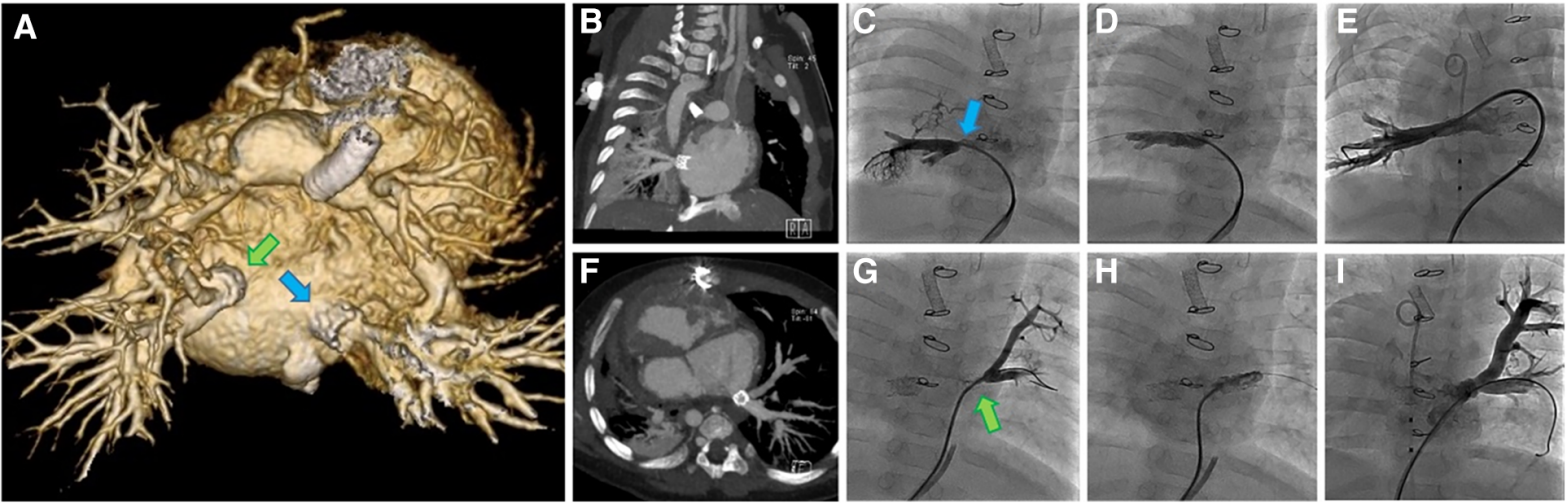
Images from a complex case undergoing implantation of multiple IBS Angel stents at our center (case 1). (A) Posterior view of 3D CT 7 weeks post stenting showing PDA stent, banded branch pulmonary arteries and IBS Angle stents (arrows) in the proximal right (RPV) and left pulmonary veins (LPV). (B) RPV 7 weeks post hybrid IBS Angel implantation, (C) RPV recurrent stenosis at 2 months (arrow), (D) RPV following implantation of a second stent at 2 months, and (E) RPV angiogram after serial reinterventions. (F) Axial CT of LPV 7 weeks post hybrid IBS Angel implantation, (G) LPV recurrent stenosis at 2 months (arrow), (H) LPV implantation of a second stent at 2 months, (I) LPV angiogram after serial reinterventions.
